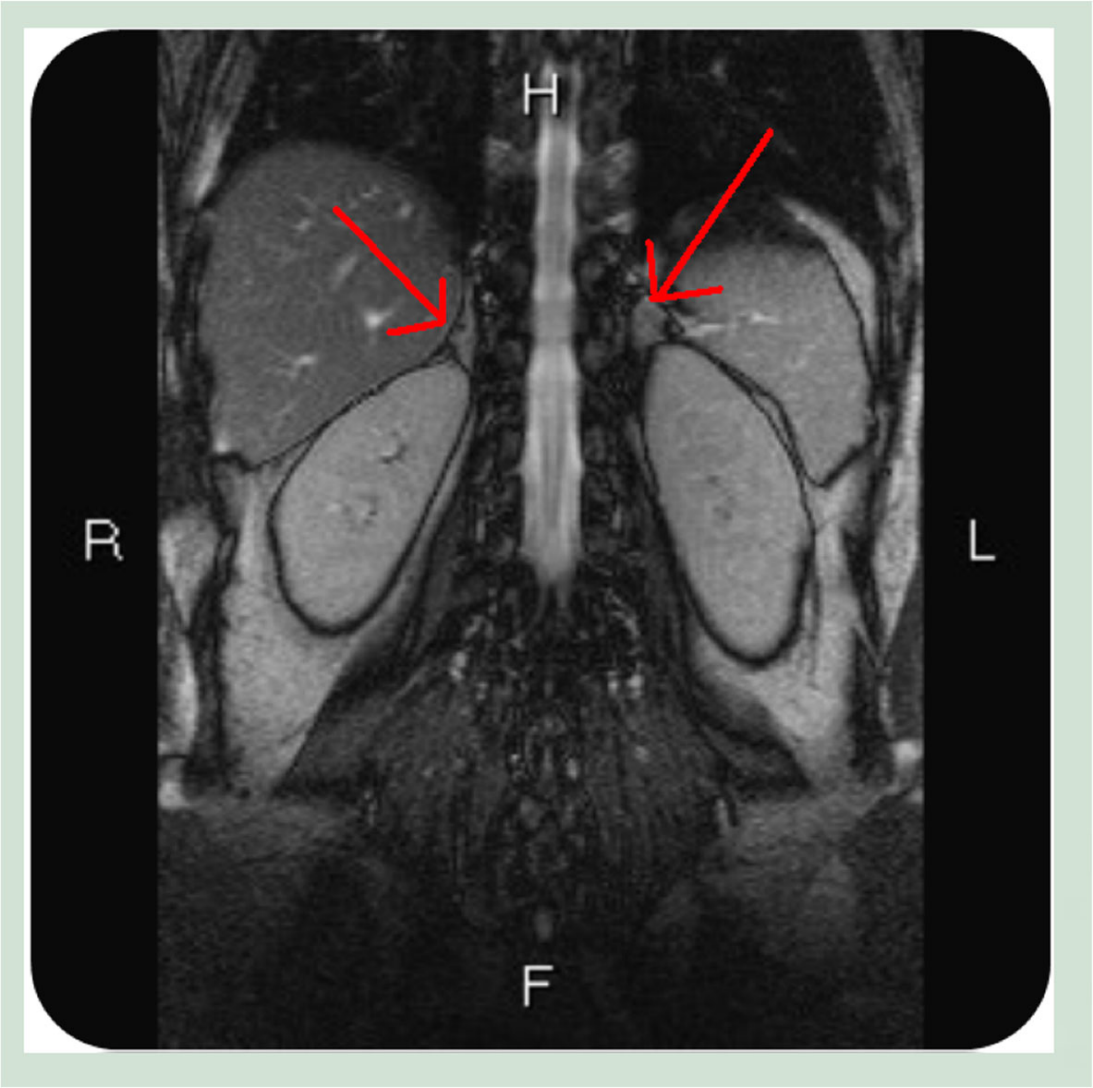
Abdominal magnetic resonance imaging revealed the relatively small size of the bilateral adrenal gland (arrow)
